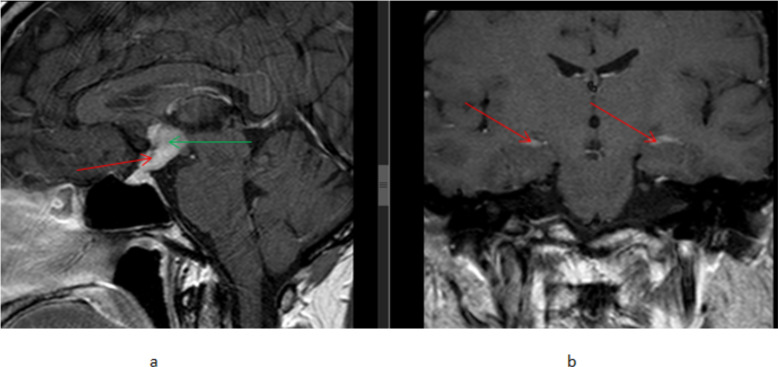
T1 brain MRI with gadolinium contrast, (a) sagittal plane showing marked mass-like thickening and diffuse gadolinium enhancement of the pituitary stalk (red arrow) and optic chiasm/hypothalamus (green arrow) (b) coronal view showing diffuse bilateral leptomeningeal thickening and gadolinium enhancement in bilateral medial temporal lobes (red arrows).
